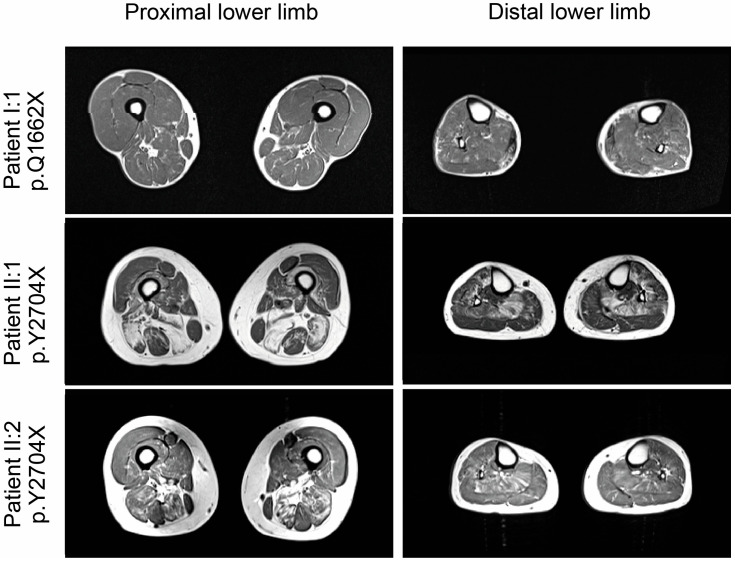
Muscle imaging findings in lower extremities.T1-weighted muscle MRI revealed no major alterations in patient I:1 (p.Q1662X), and moderate fatty degenerative changes in posterior thigh muscles (especially semimembranosus and biceps femoris), soleus and anterior tibial muscles in patients II.2 and II:1 (both p.Y2704), which were pronounced in the older patient (II:1).
